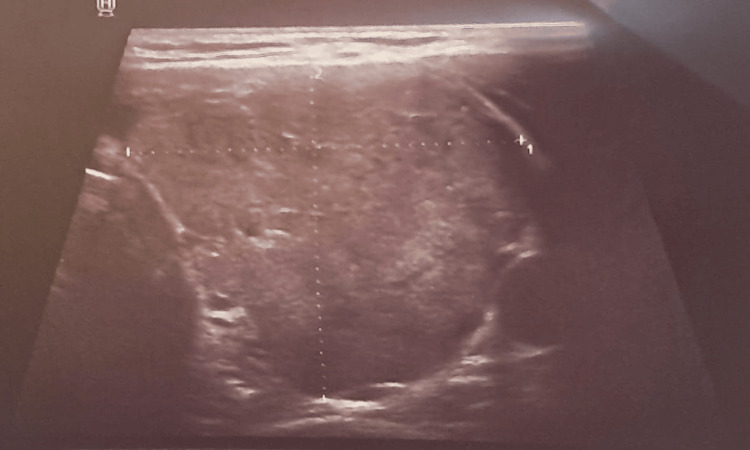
Ultrasound image of the left thyroid lobe showing a goiter involving the left thyroid gland with a heterogeneous echostructure
